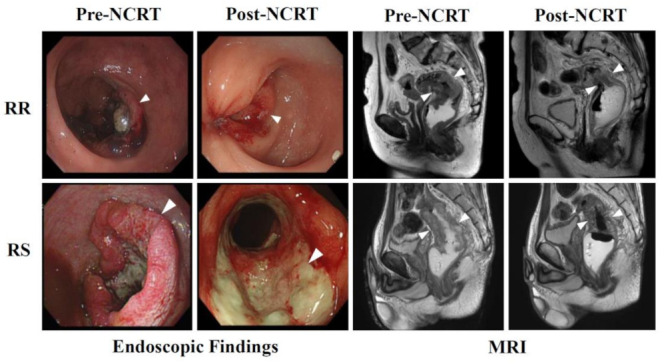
NCRTs cause differential effects in LARC patients. Endoscopic findings (left panels) and magnetic resonance images (right panels) are shown for radioresistant (RR; upper panels) and radiosensitive (RS; lower panels) patients. Pre- and Post-NCRT images are compared. White arrows indicate LARC tissues.
